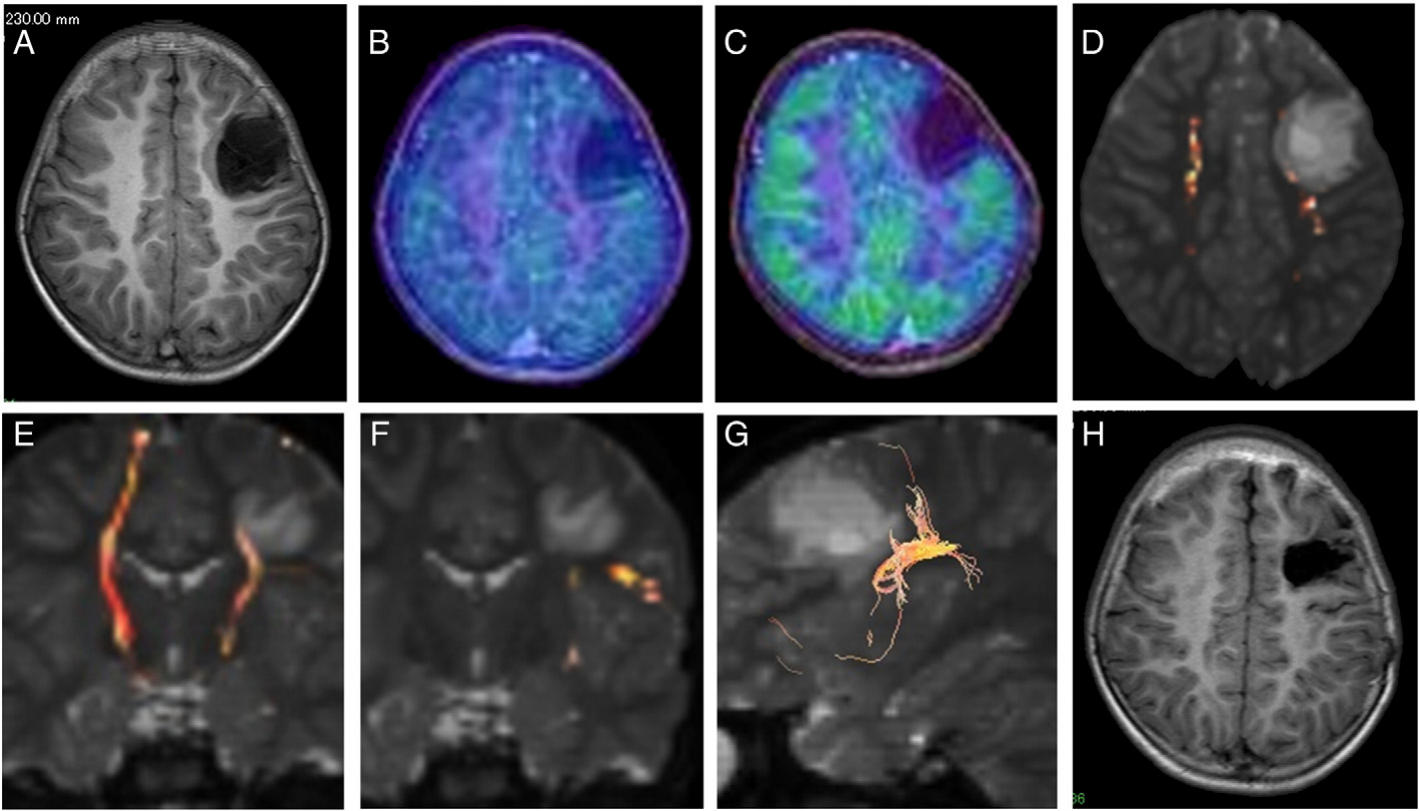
Case 2. T1WI showing a well-demarcated hypointensity mass lesion in the left frontal lobe (A). The tumor shows low uptake in MET-PET (fused with T1WI) (B) and hypo-uptake in FDG-PET (fused with T1WI) (C). Fiber tractography showing that the left pyramidal tract is located posteromediocaudal to the tumor (D and E) and that the left arcuate fasciculus is located caudolateral to the tumor (F and G). Postoperative T1WI showed the tumor to be totally resected (H).
